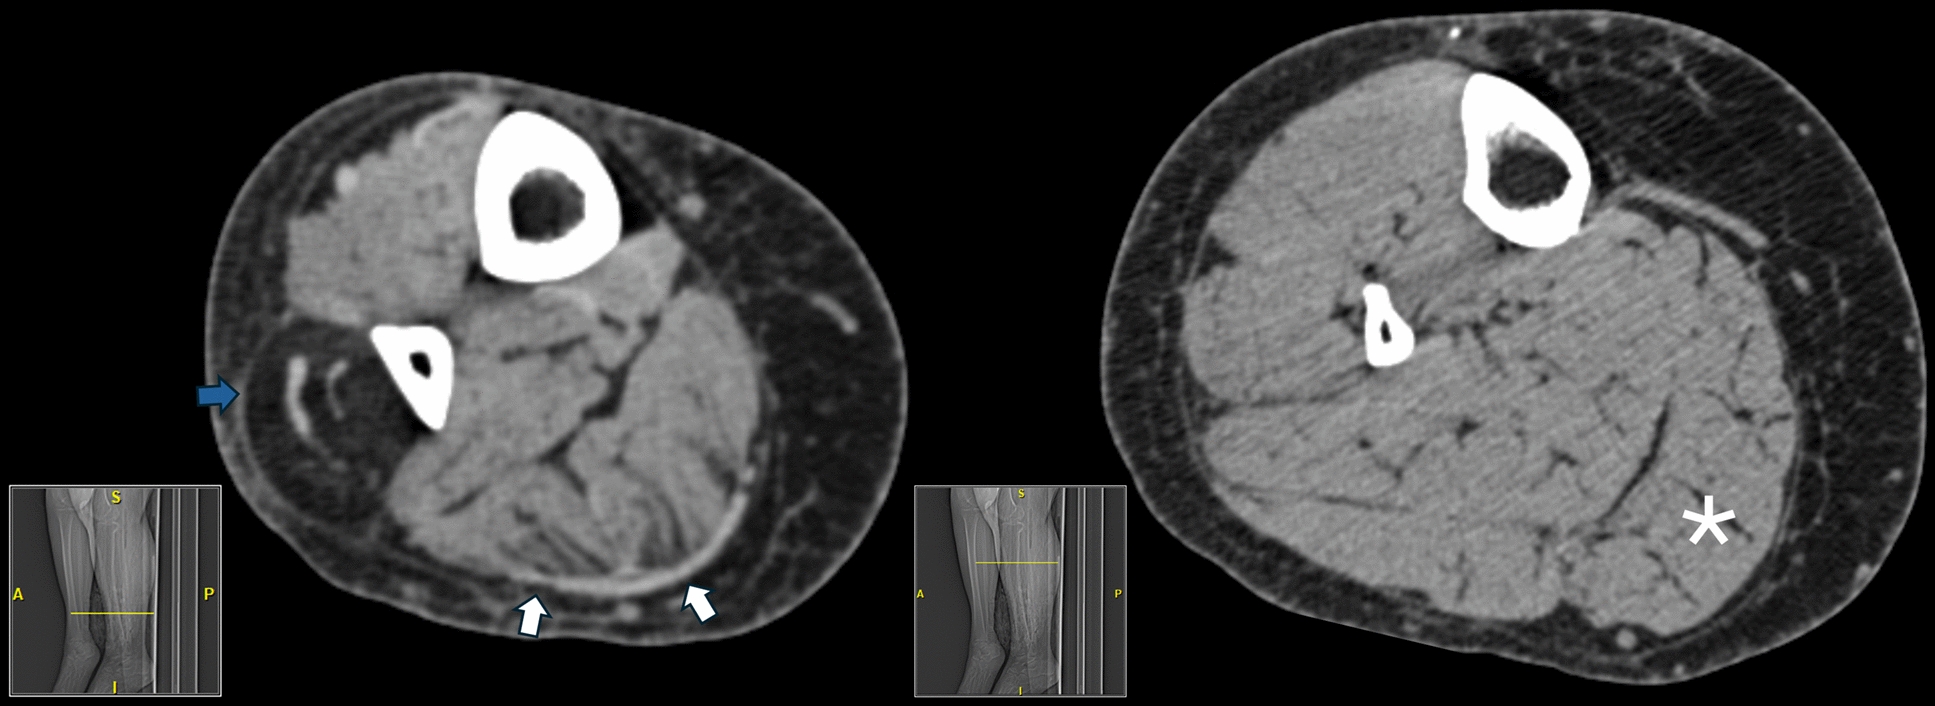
Patient 1's CT images. Non-contrast axial CT of the right lower leg (left) revealing hypodense fatty infiltration most prominently of the distal peroneal musculature (blue arrow) with mild muscular marbling of the soleus and gastrocnemius (white arrows), which is spared more proximally (right, asterisk). A = anterior; S = superior; P = posterior; I = inferior
